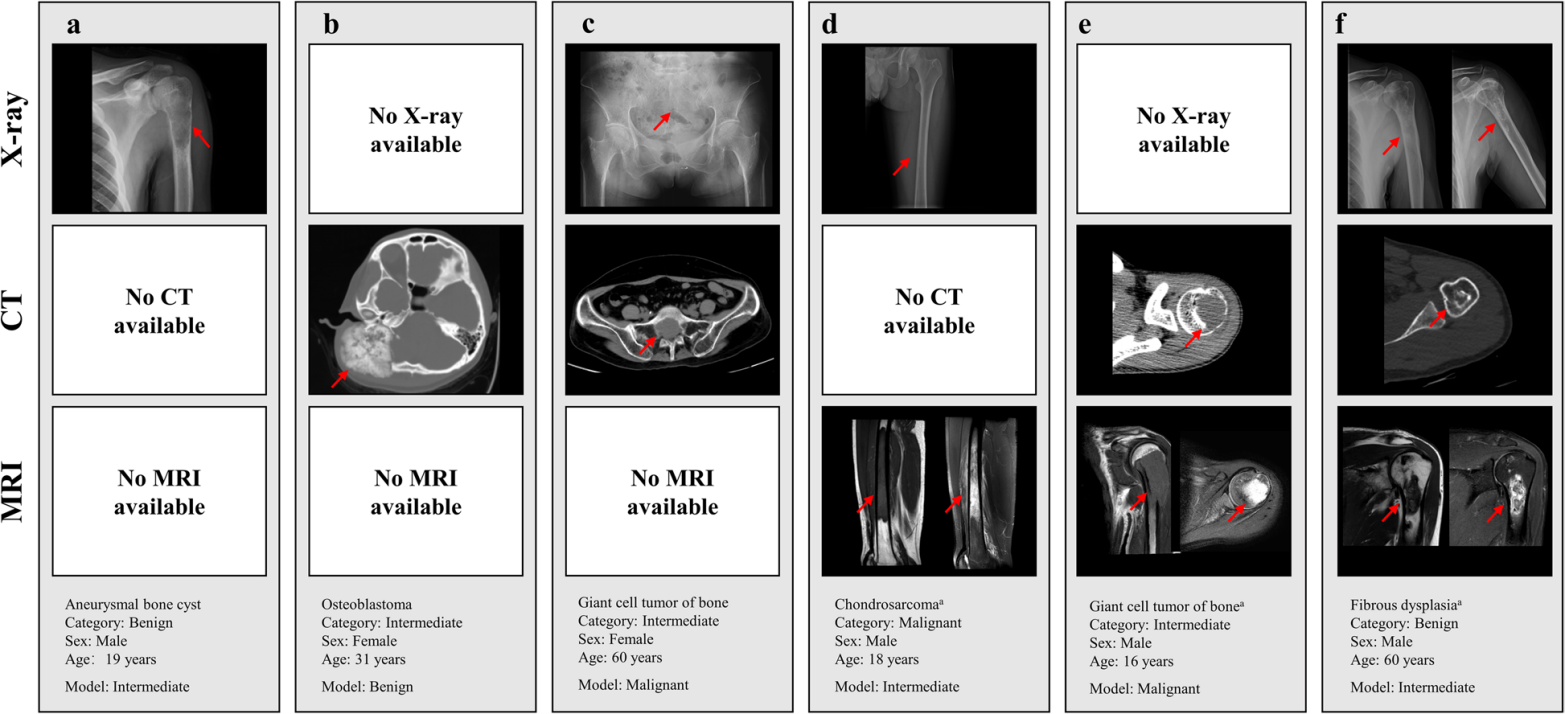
Representative examples (red arrow) incorrectly classified by the PBTC-TransNet fusion model on the external test set. ^a^T2-weighted image (T2WI) with fat-suppression is provided to the model when T2WI was not available. PBTC-TransNet, Primary Bone Tumor Classification Transformer Network
